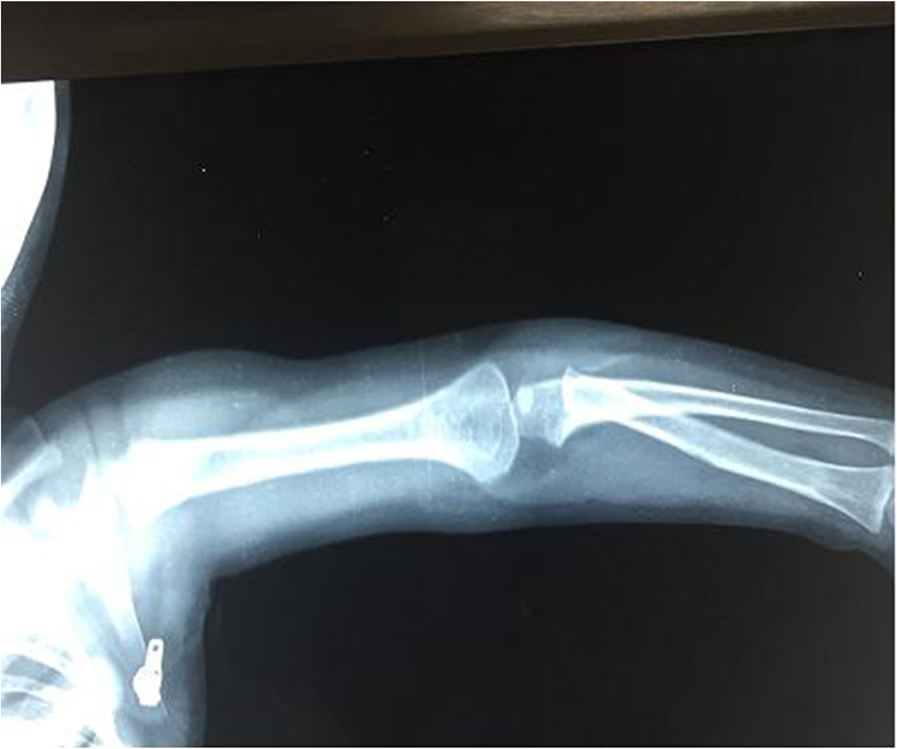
A follow-up X-ray with complete disappearance of the lytic lesion
